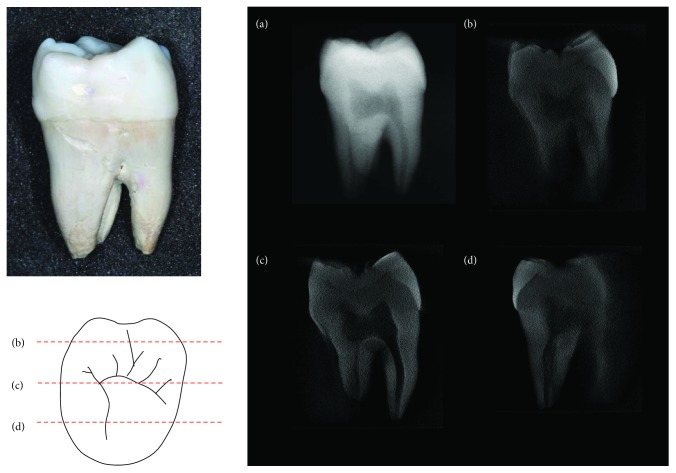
(a) Periapical radiology image of the third molar and (b–d) the reconstructed slice images at different depths from the sensor surface.
